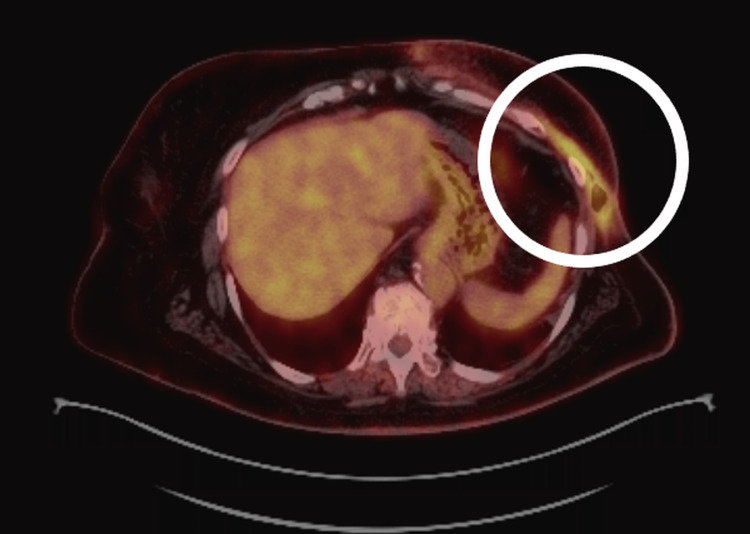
PET/CT imaging during systemic therapy (July 2024). Axial PET/CT image demonstrating focal increased fluorodeoxyglucose uptake in the left anterior chest wall (circled), consistent with persistent localized disease. The metabolic activity is increased compared to prior imaging (maximum standardized uptake value (SUVmax) = approximately 5.7), without evidence of distant metastatic spread.
